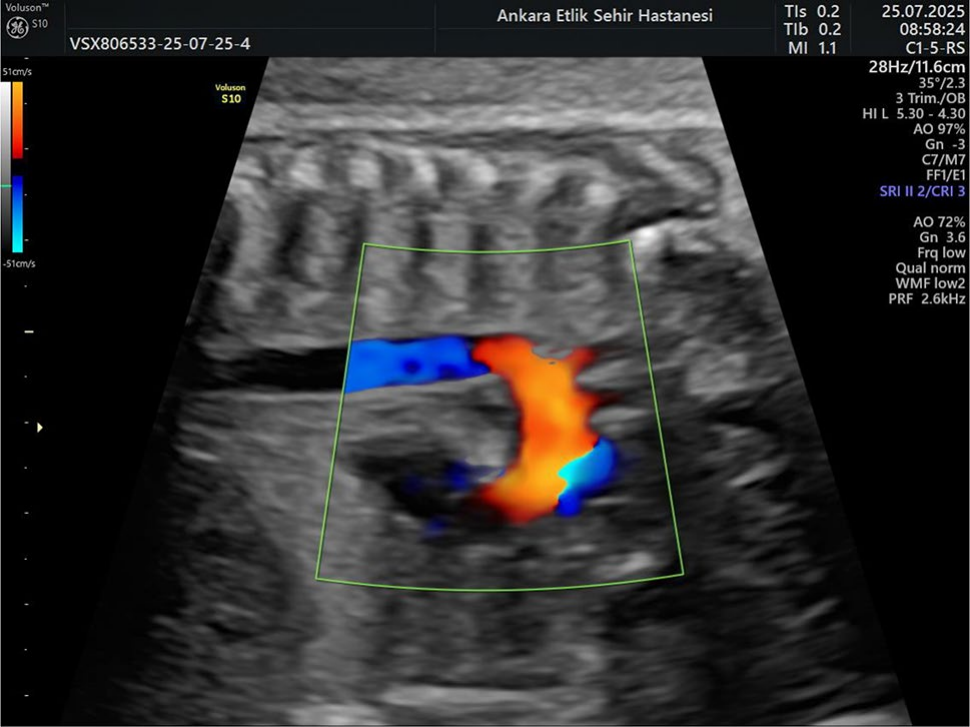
Sagittal color Doppler ultrasound image of the fetal aortic isthmus showing blood flow between the aortic arch and descending aorta
